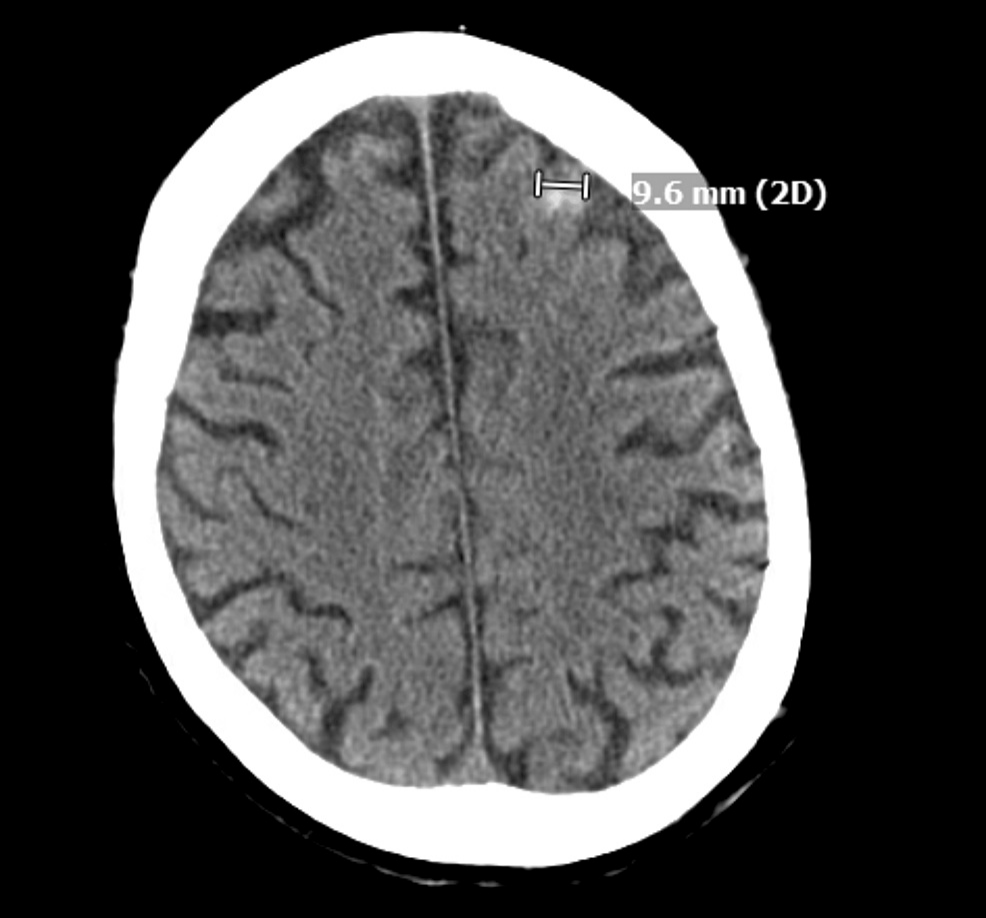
CT scan of the head following stroke incident showing a focal area of left frontal acute parenchymal hemorrhage
